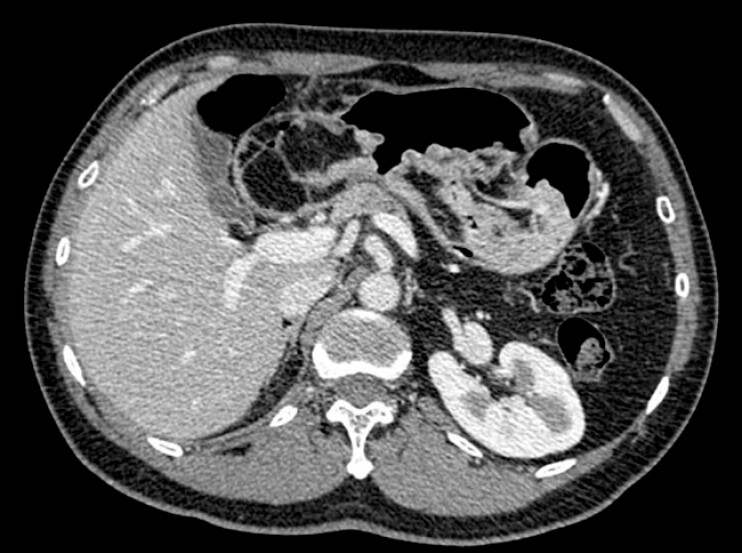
Contrast-enhanced computed tomography showed fat-dense nodules.
